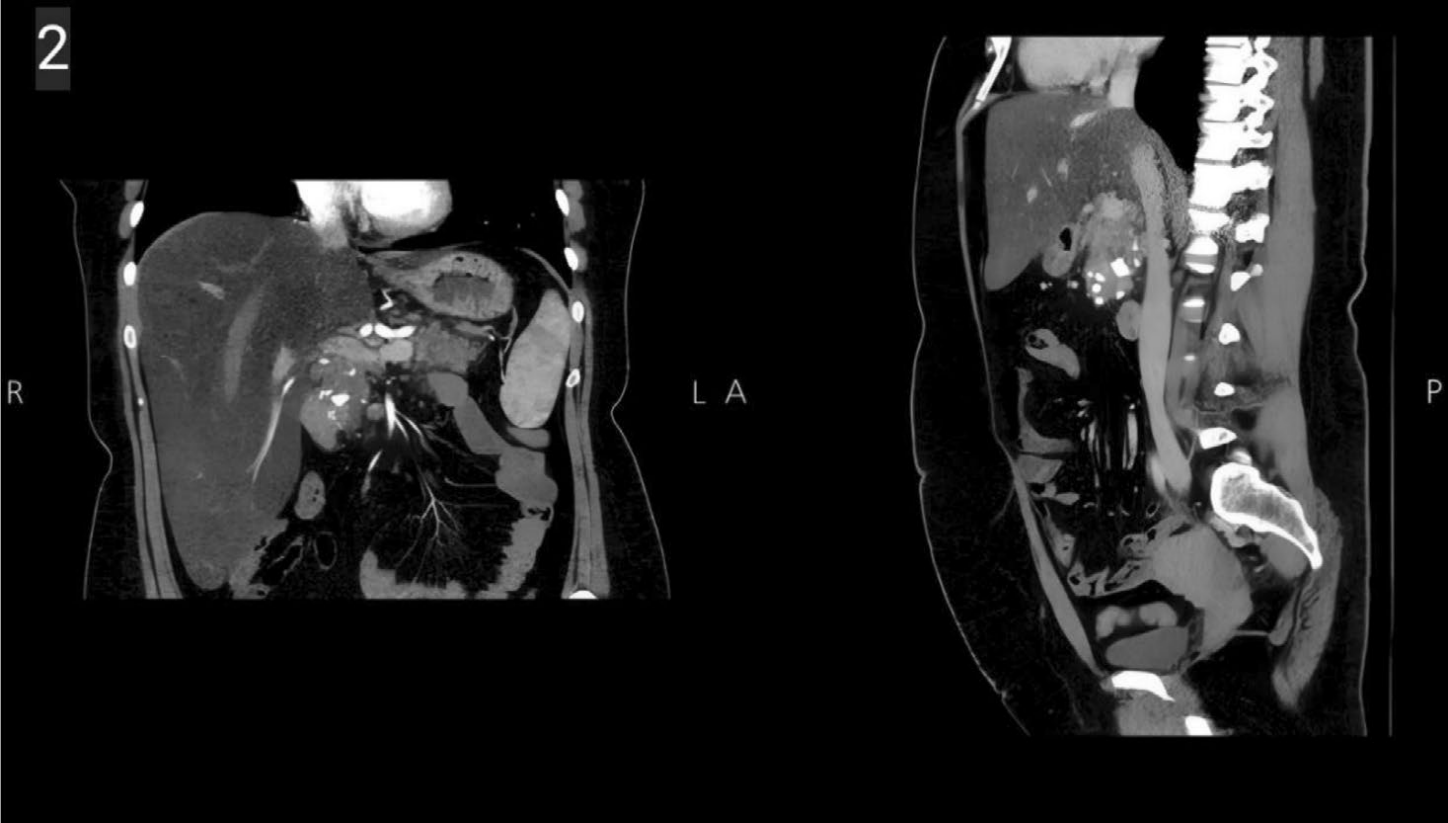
Sagittal and coronal abdominal CT scan images show ductal dilation, multiple intraductal calculi, parenchymal calcifications, and mild peripancreatic fat stranding.
